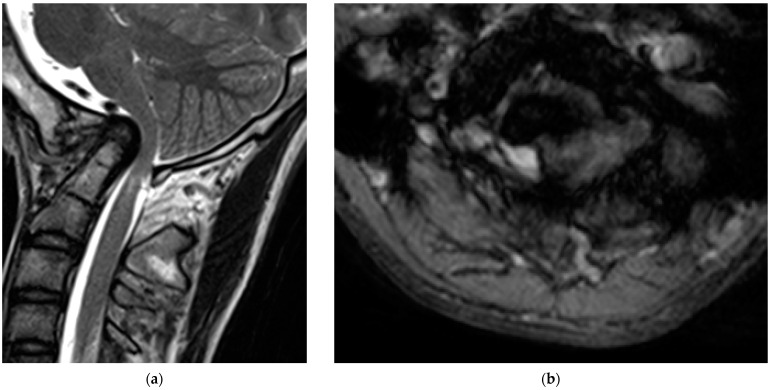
Preoperative magnetic resonance images: (a) sagittal; (b) axial view of the T2-weighted images.
